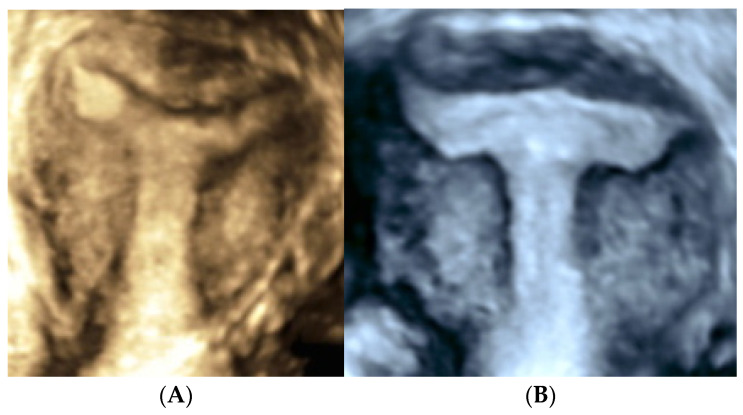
(A) Transvaginal three-dimensional ultrasound images of a Y-shaped uterus. (B) Transvaginal three-dimensional ultrasound images of a T-shaped uterus.
